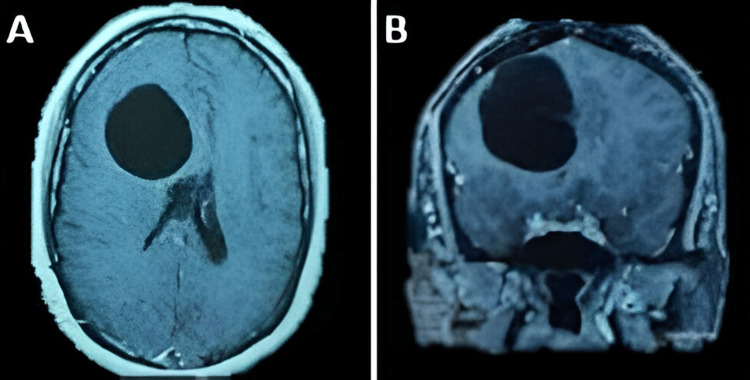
Right frontal lobulated giant neurocysticercosis. Brain MRI shows giant neurocysticercosis at the right frontal level, multipartitioned in the axial (A) and coronal (B) planes MRI: magnetic resonance imaging
